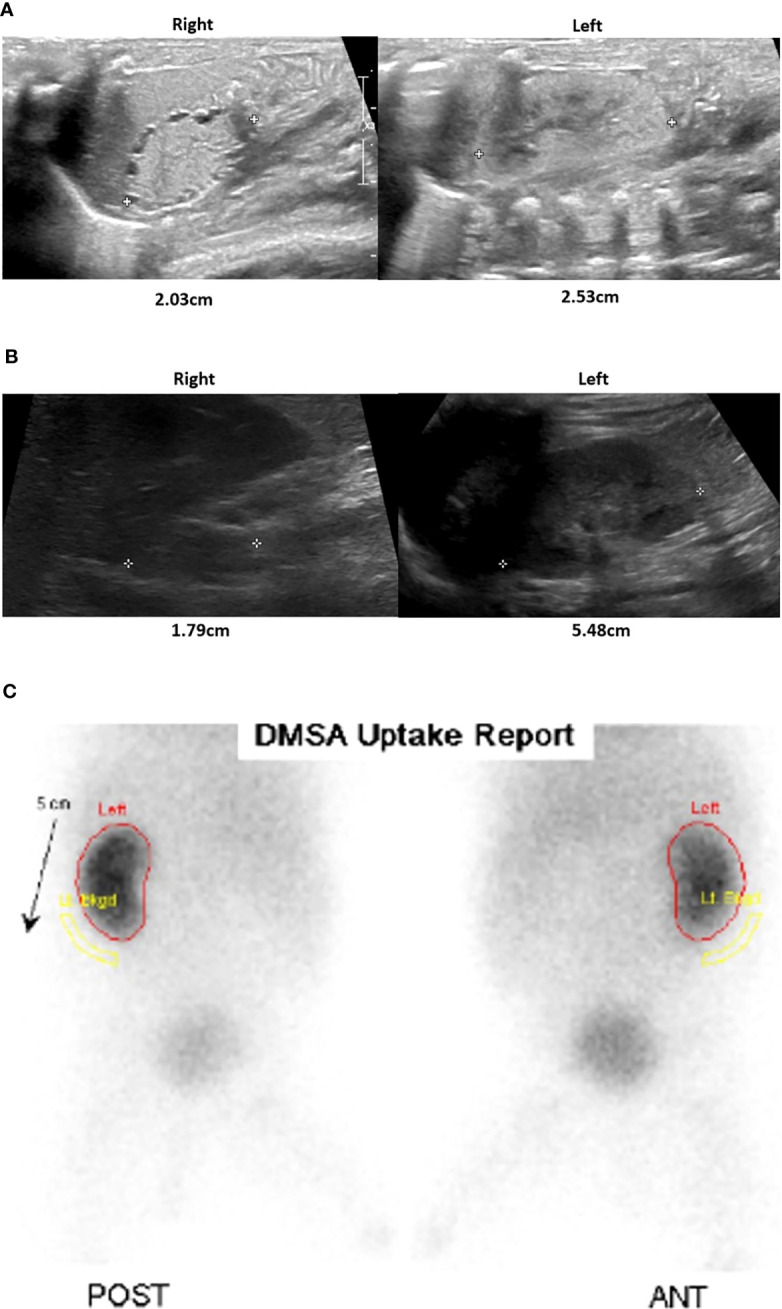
Renal and adrenal ultrasonography and renal scan. After birth, the parenchymal echogenicity of both the kidneys was increased, showing an undifferentiated corticomedullary junction and tiny cortical cysts. The right kidney was smaller than the left kidney (A). At 20 months of age, there was a small right kidney, but the left kidney growth was relatively preserved, although cortical echogenicity remains increased (B). In the Tc-99m Dimercaptosuccinic acid (DMSA) scan obtained at 6 months, the right kidney uptake is barely visible, indicating nonfunction, while the left kidney function appears unremarkable (C).
